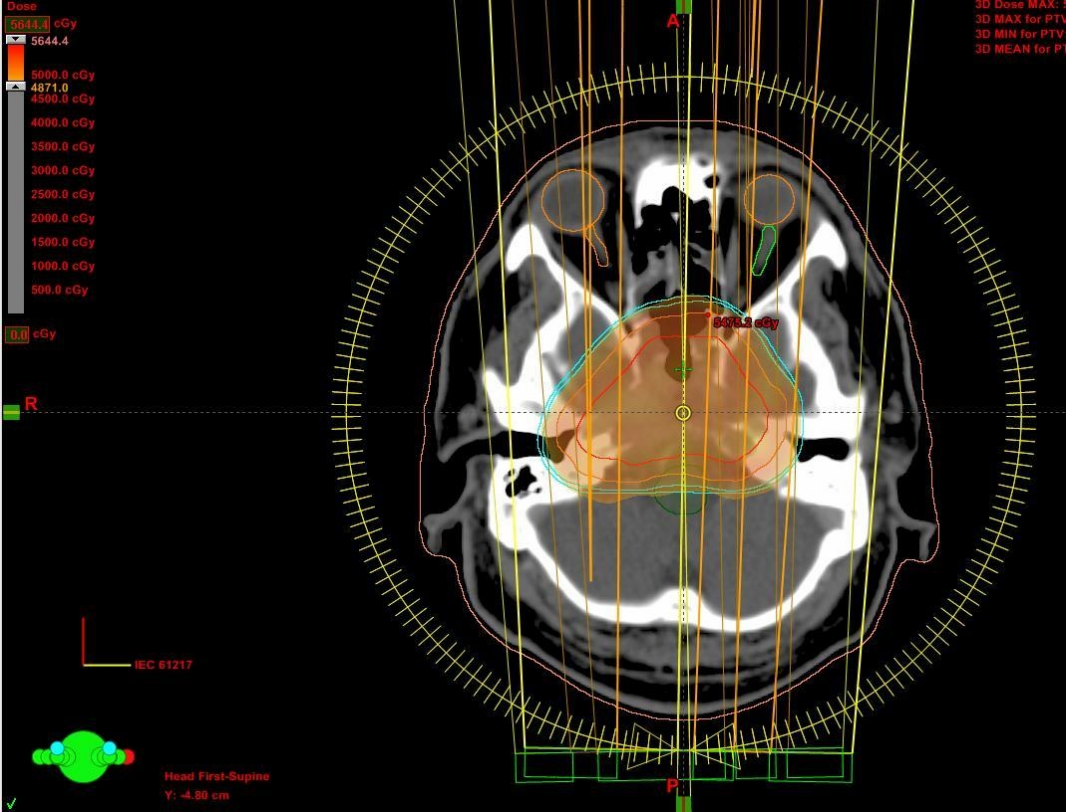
Axial planning computed tomography slice showing typical dose color-wash and dose distribution of intensity modulated radiotherapy by the RapidArc technique
